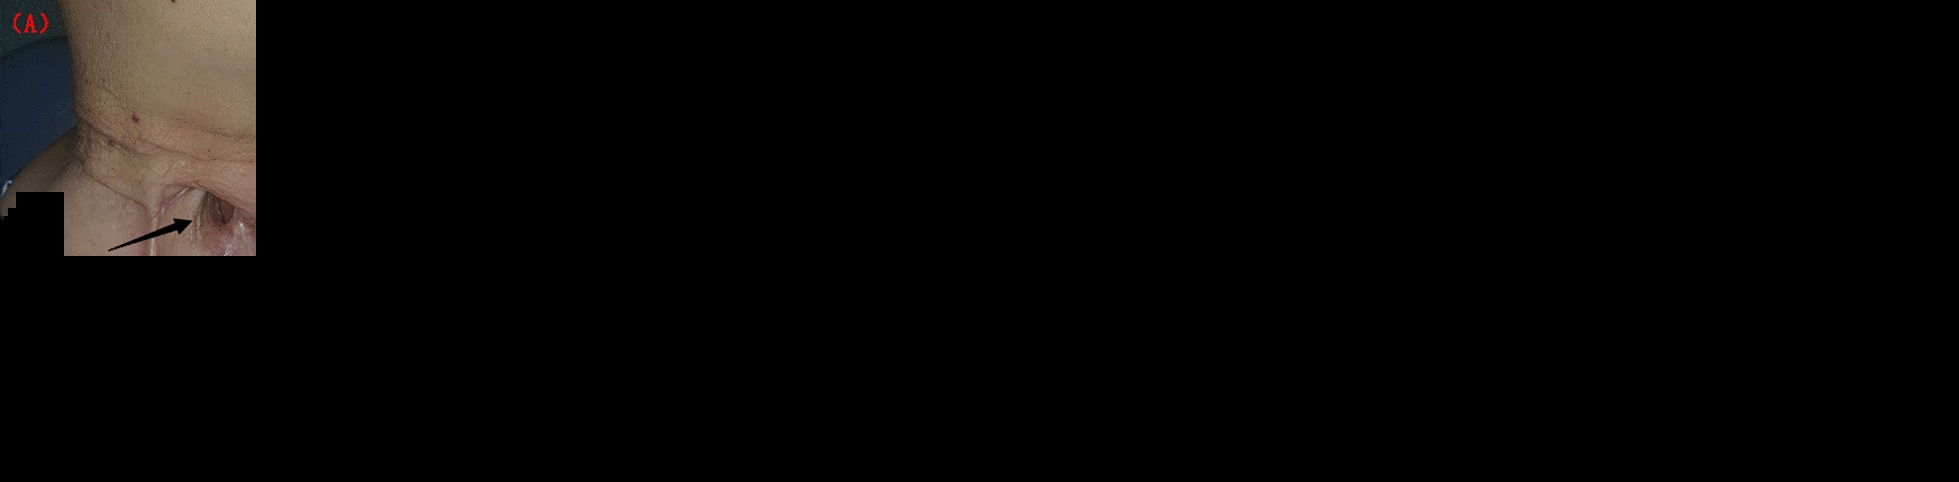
Postoperative follow-up. a The TAAP flap was in good condition (black arrow) under direct vision after the first stage tracheal reconstruction. b The donor site was well after operation. c, d CT showed the trachea after the second stage tracheal reconstruction with a local flap. The blue and red arrow indicates the pedicled TAAP flap, the green arrow indicates the local flap
